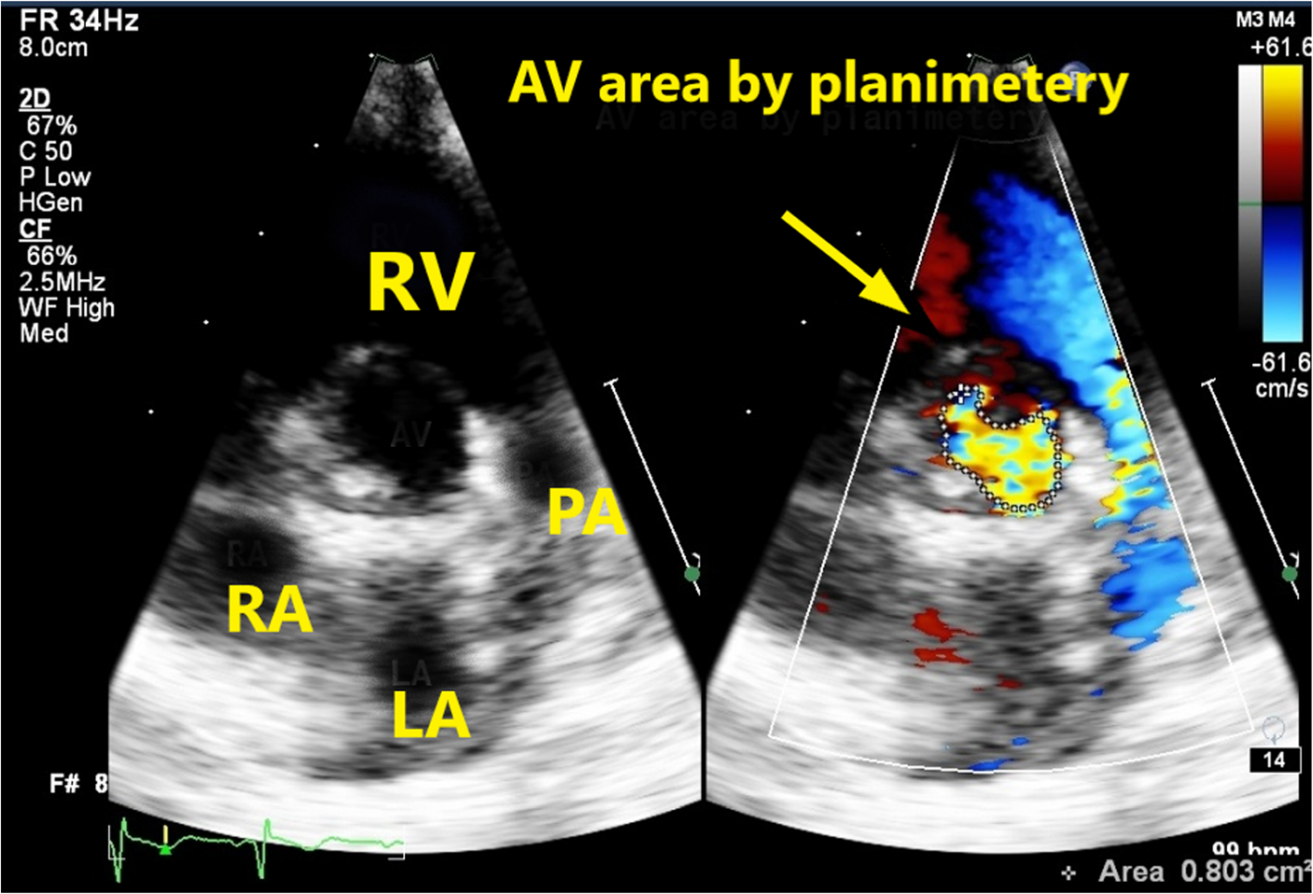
AVA was measured by direct planimetery in parasternal short axis view in patient no. 9
